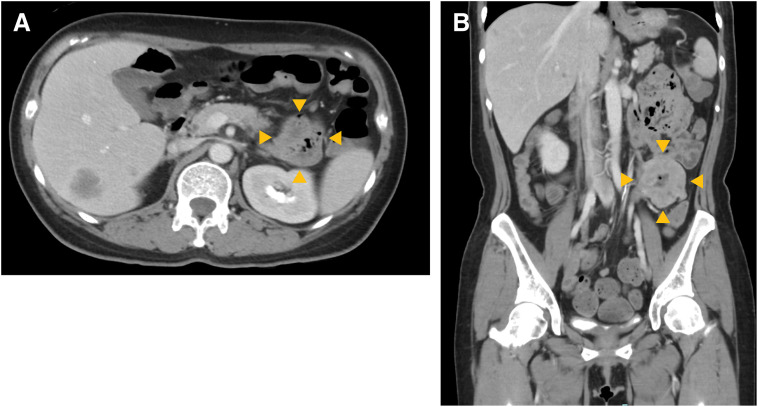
Findings of contrast-enhanced abdominal CT scan. Contrast-enhanced abdominal CT: (A) axial and (B) sagittal views. Segmental thickening of the small intestinal wall is noted (arrowheads), and hepatic metastases are also evident on the axial view.
